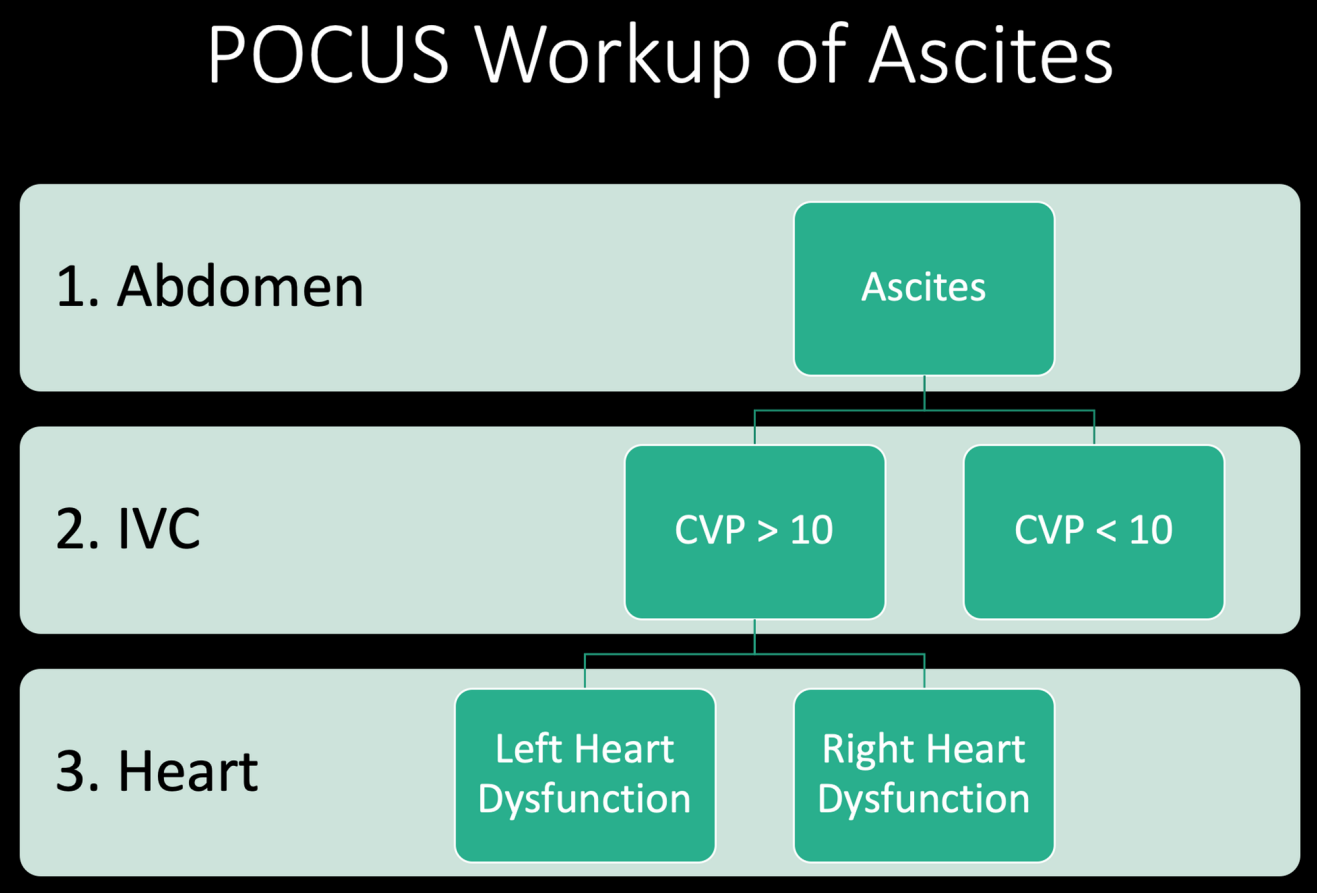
Framework for the workup of ascites. Step 1: confirm ascites. Step 2: evaluate the IVC. Step 3: If the CVP is elevated evaluate the heart. CVP, central venous pressure; IVC, inferior vena cava; POCUS, point-of-care ultrasound
